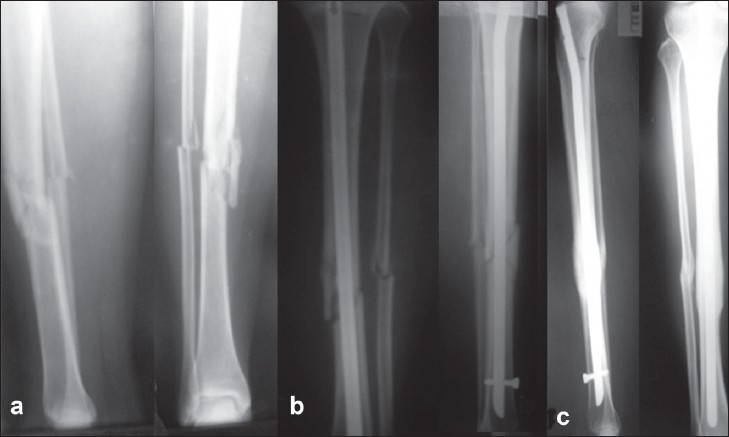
(a) X-ray of right leg lateral and anteroposterior views showing a fracture of both bones of leg, middle third with a butterfly fragment. (b) 6 weeks postoperative (anteroposterior and lateral) radiographs showing early healing, (c) radiograph showing good consolidation at 1 year follow-up.
